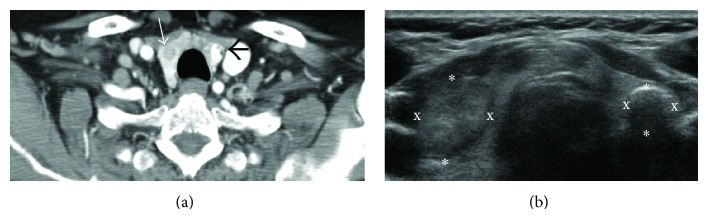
(a) Contrast enhanced computed tomography (CT) of the thorax. Thin, white arrow indicates the largest nodule in the mid pole of the right thyroid lobe, measuring 1.6 × 1.2 × 1.4 cm. Black, thick arrow demonstrates a calcified nodule in the mid-to-lower left thyroid lobe measuring 1.1 × 0.9 × 0.9 cm. There are multiple scattered calcified nodules noted throughout the thyroid gland. (b) Axial ultrasound of the thyroid gland. There is evidence of a solid isoechoic nodule in the right thyroid lobe measuring 1.6 × 1.2 × 1.4 cm and a peripherally calcified nodule in the left thyroid lobe measuring 1.1 × 0.9 × 0.9 cm.
